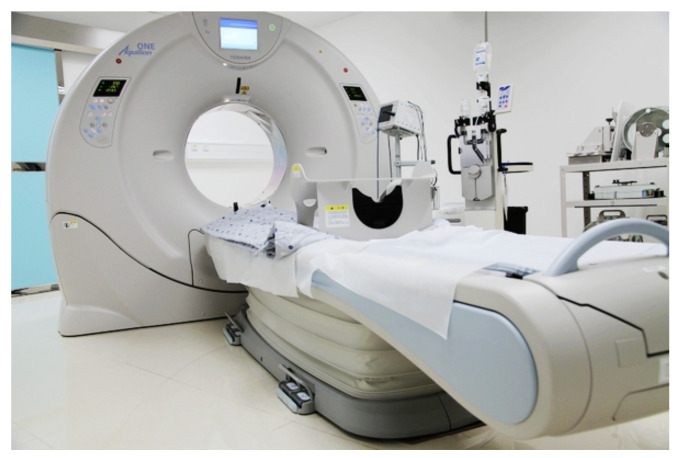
Toshiba Aquilion ONE 640-slice dynamic organ volume CT (taken in the Radiology Department, the Sixth Affiliated Hospital of Sun Yat-sen University).
